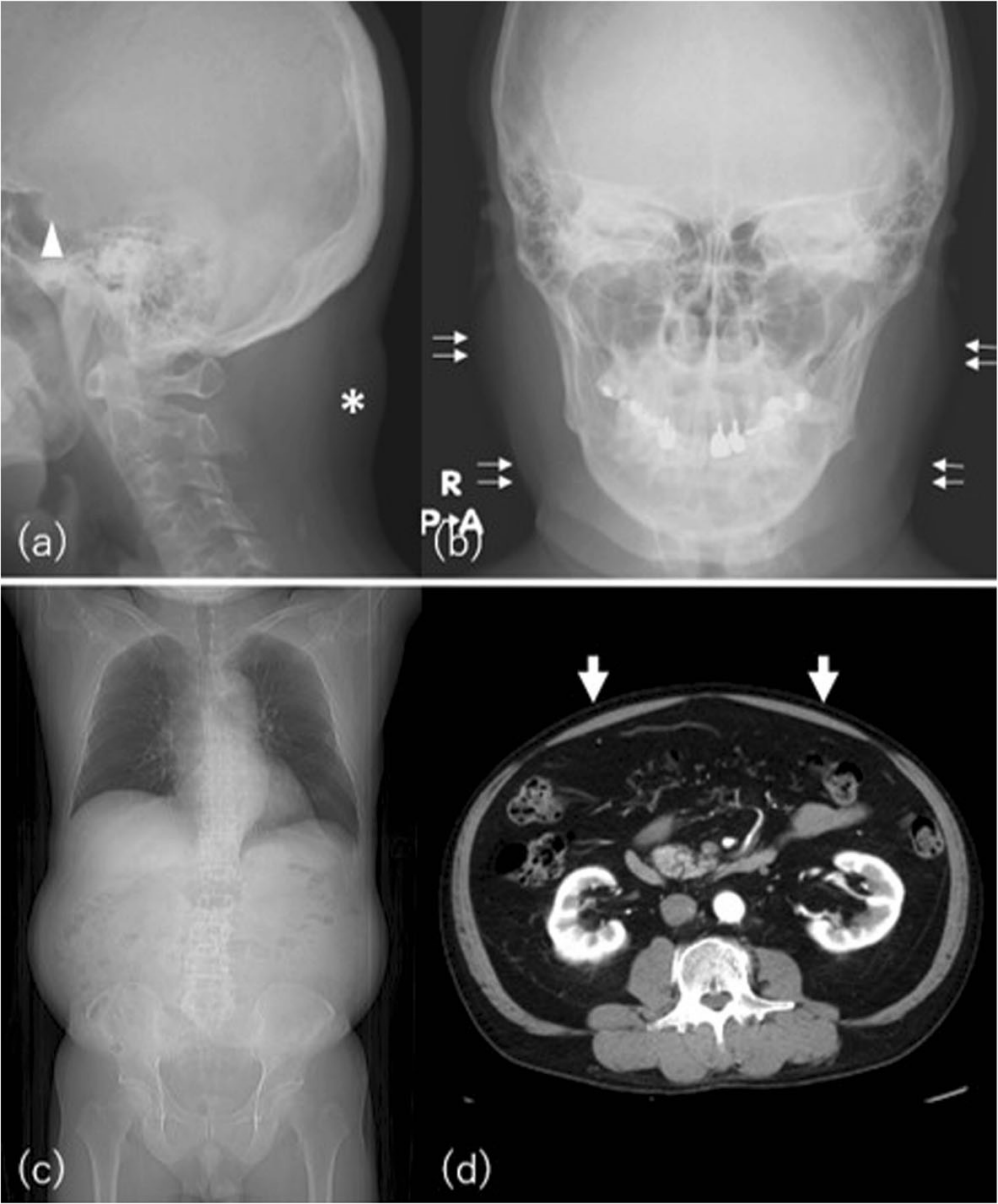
A 52-year-old man with Cushing disease. a Lateral skull radiography shows “buffalo hump” on the posterior neck (asterisk). Note the enlarged sella due to pituitary macroadenoma (arrowhead). b Frontal skull radiograph shows a rounded shape of the face, “moon face” (double arrows). c CT scanogram shows characteristic central obesity. d CT image at the level of bilateral renal hilum shows marked visceral fat deposition, in contrast to subcutaneous fat thinning (boxed arrows)
